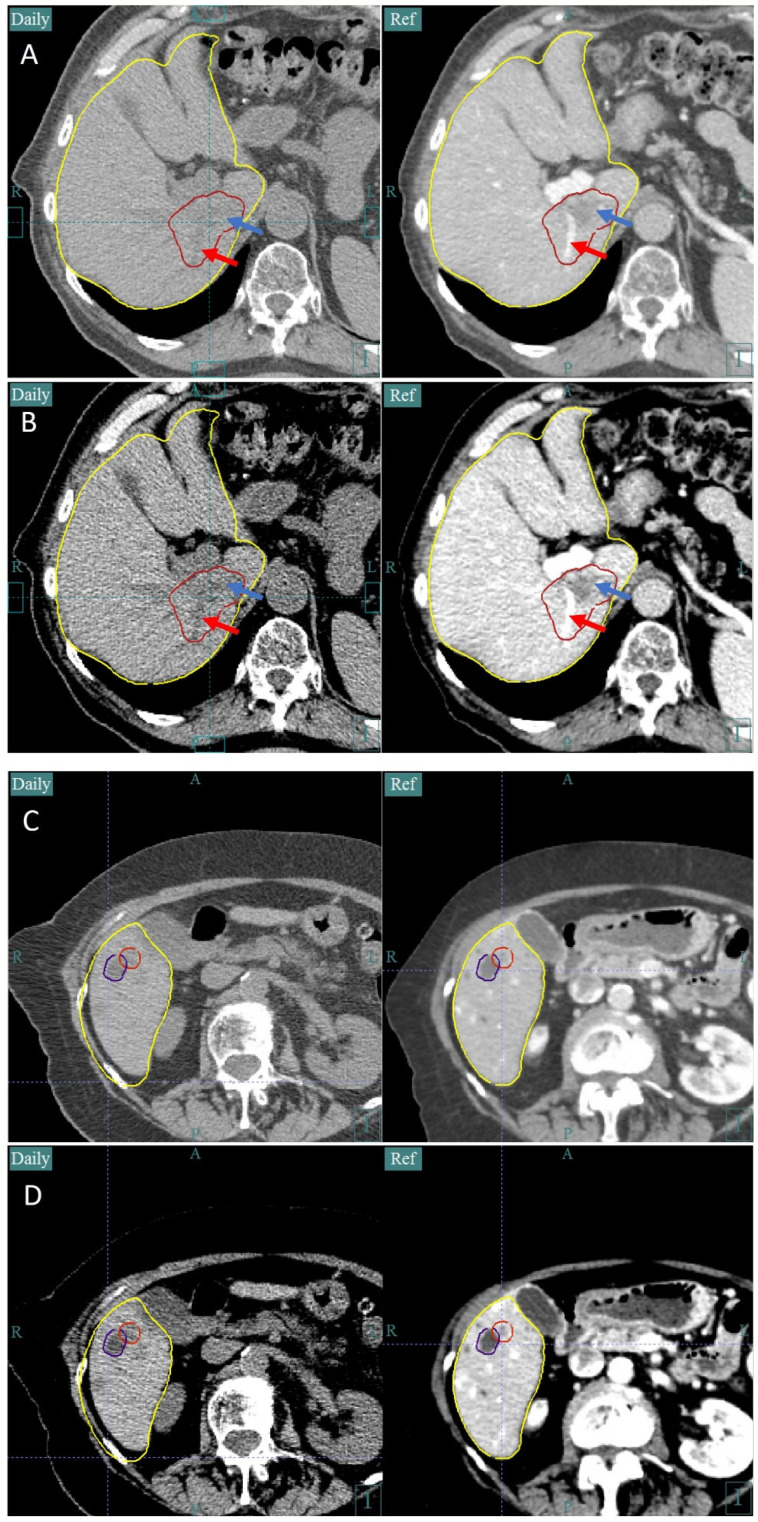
This figure demonstrates the ability to visualize the CTOR features within the liver necessary to accurately align with the GTV. In the left column are the CTOR images and in the right are the planning CT images. For the patient in (A), the liver shape (yellow contour), blood vessels, liver fissures in the vicinity of the tumor can be used to align to the GTV (red contour). Additionally, the tumor itself is somewhat visible (blue arrow), along with a blood vessel within the liver (red arrow). Using a different window and level (B) can improve the visualization of the tumor and interior blood vessel. In (C), the tumor itself is difficult to distinguish from normal liver, but a cyst (purple contour) right next to the tumor (red contour), along with the inferior liver shape, can be used to achieve accurate alignment. With a different window and level (D), the cyst becomes easier to distinguish and there is some distinction between the tumor and normal liver. In (A,C), a window level of 400/800 HU is used and, in (B,D), a window level of 180/950 HU is used.
